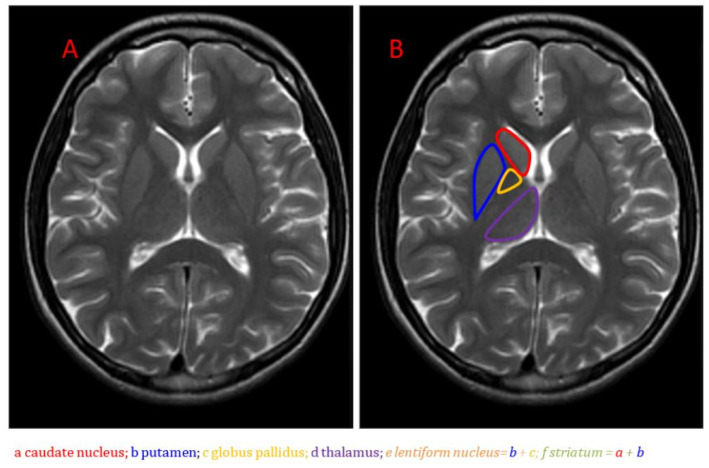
Anatomical MRI of the basal ganglia in the axial plane on T2-weighted imaging: (A) Unlabeled image; (B) Image with caudate nucleus, putamen, globus pallidus, and thalamus labeled [7].
